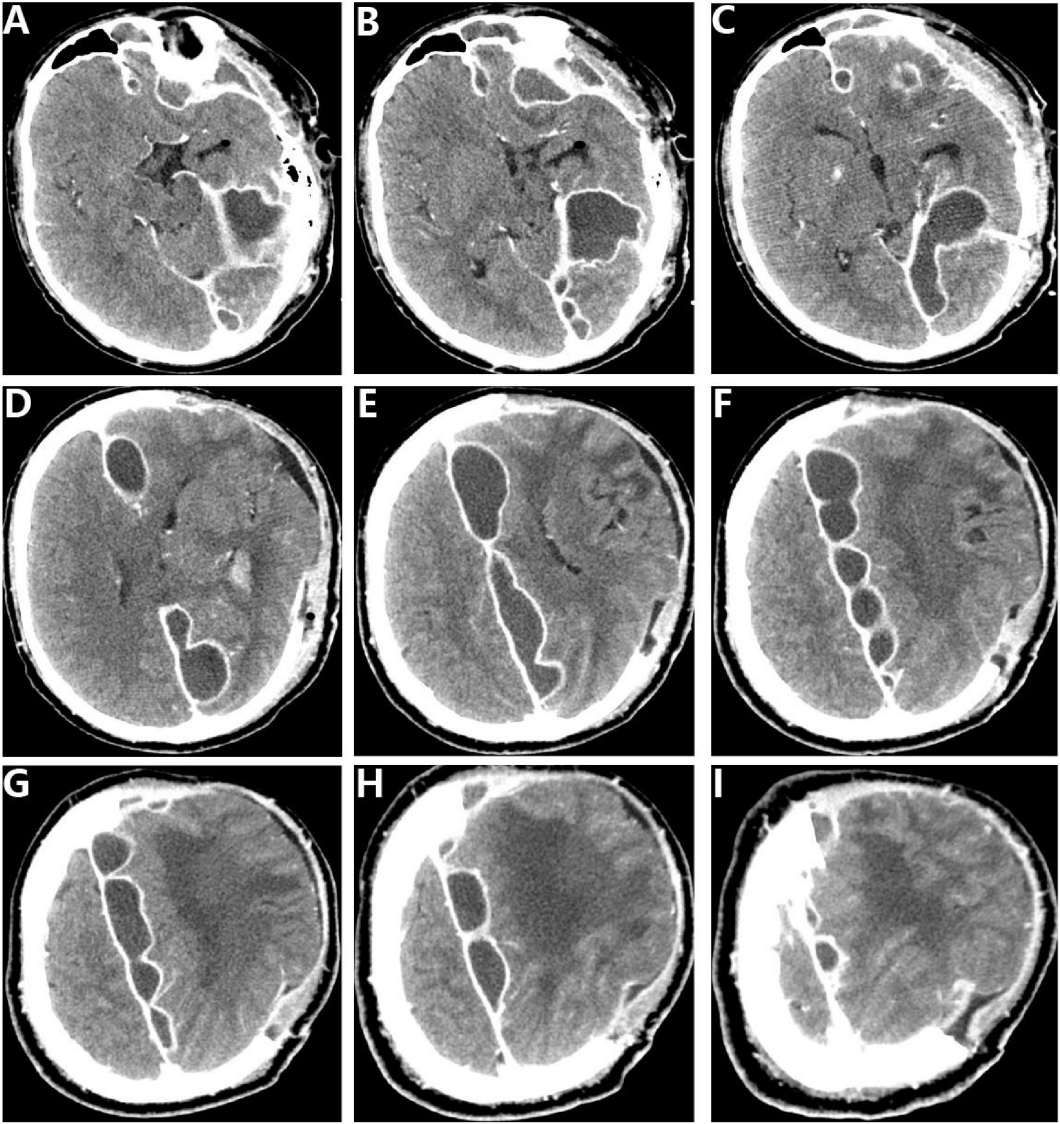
Head enhanced CT prior to neuroendoscopic treatment of the patient (preoperative CT). (A–I) Preoperative head CT images of the patient showing the condition of brain abscesses.
